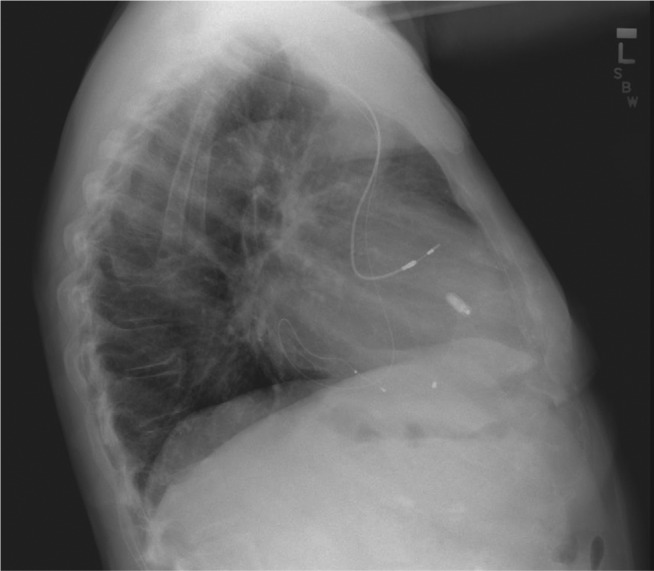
Lateral chest X-ray following initial lead extraction and leadless pacemaker insertion. Shown in the image is a right atrial lead, a CS lead, and a Micra™ pacemaker (Medtronic, Minneapolis, MN, USA).
